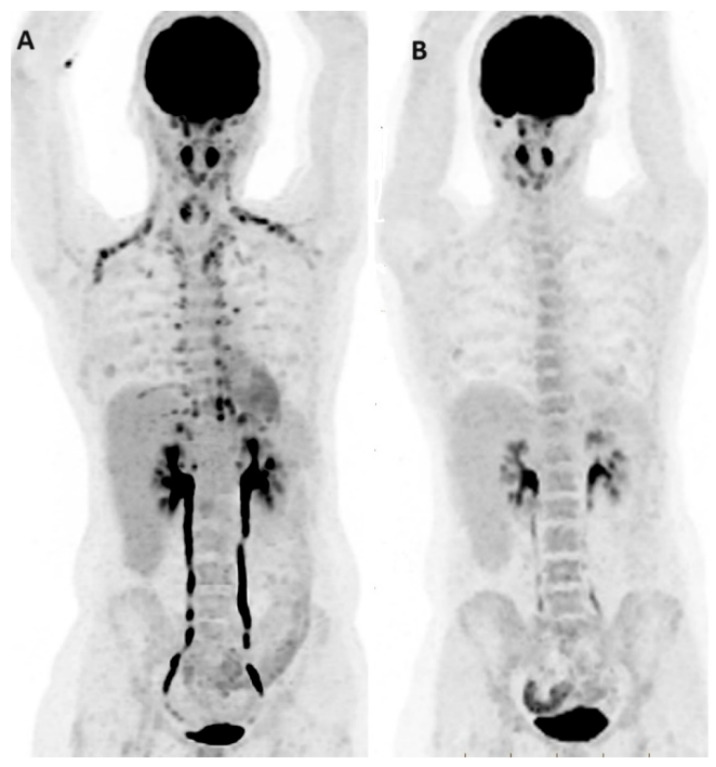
Physiologic brown fat uptake. MIP FDG PET/CT imaging (A) demonstrates extensive brown fat uptake, leading to a technically limited, non-diagnostic study in a 45-year-old patient. The patient returned for a repeat PET scan (B) on another date post-administration of beta-blockers.
